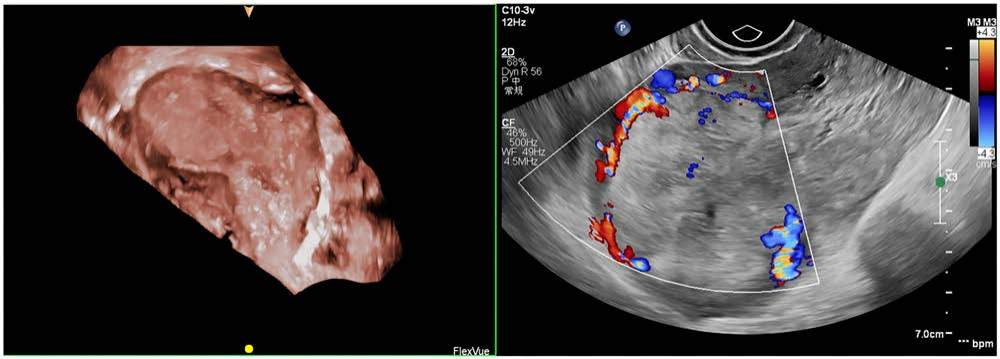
Three-dimensional ultrasound images of uterine horn lesions.
